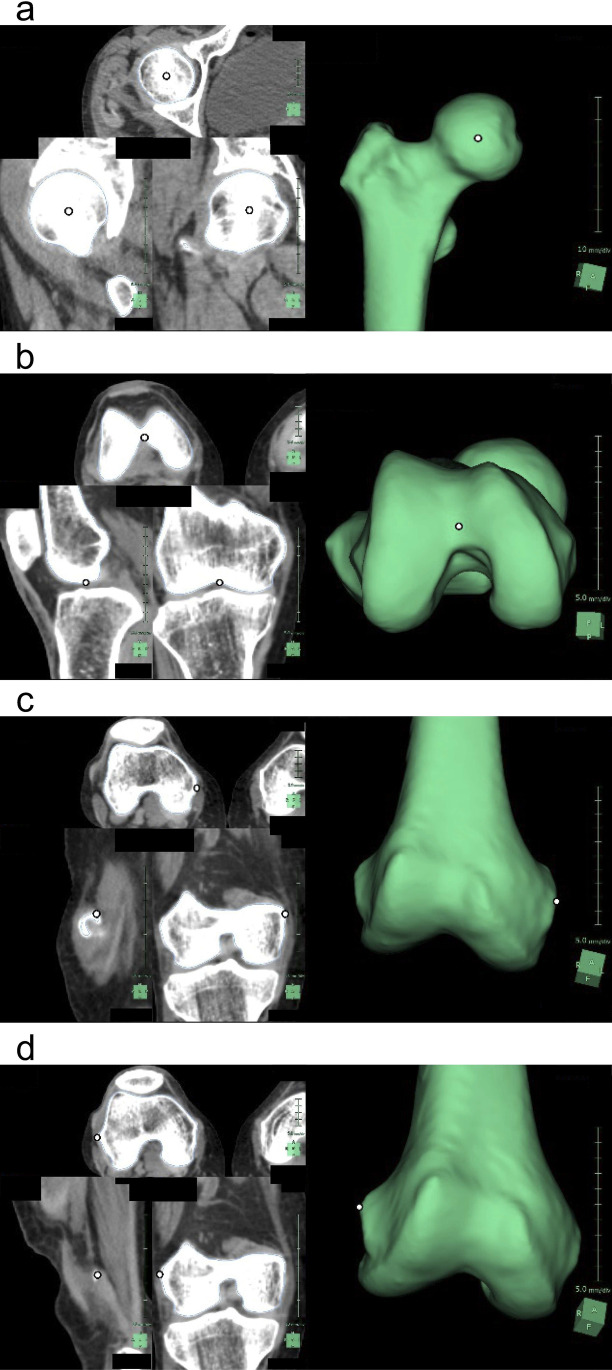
Four points where manual marking are necessary (each picture shows one point in the horizontal, coronal, and sagittal planes and the three-dimensional reconstruction of the computed tomography images). a Center of the femoral head: the central point of the femoral head. b Intercondylar notch: posterior 1/4 point on the midline of the recess located between the medial and lateral condyles on the bottom surface of the lower end of the femur. c Medial epicondyle: the most medial point of the medial condyle. d Lateral epicondyle: the most lateral point of the lateral condyle
